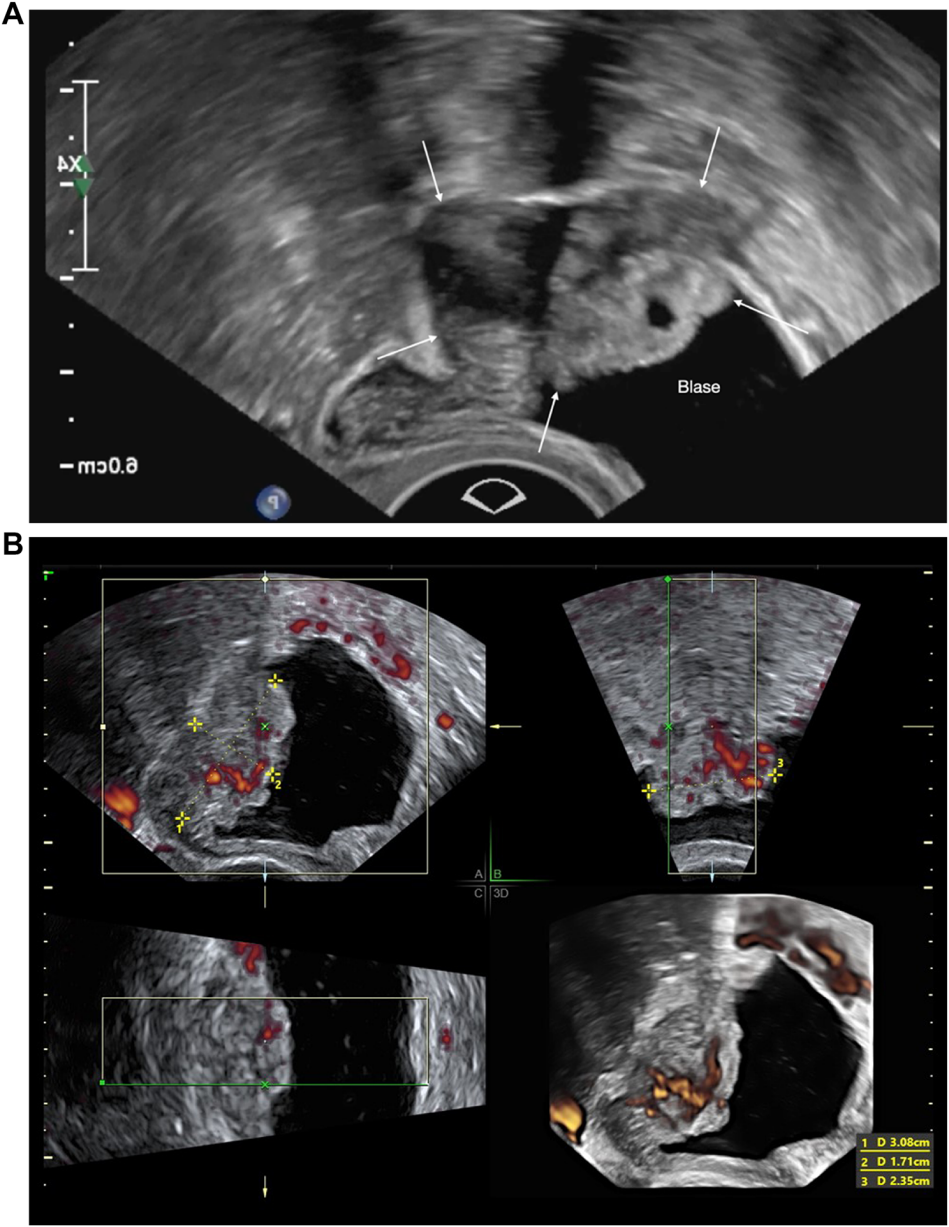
a Sonographic view of DE of the bladder (#Enzian(u)FB), presenting a full thickness defect at the bladder dome by a large inhomogeneous deep endometriosis nodule. b 3D demonstration of a severe bladder endometriosis (30.8 × 17.1 × 23.5 mm) originating from the posterior bladder wall (#Enzian(u)FB). A–C Multiplanar glass body demonstration (grey scale + color Doppler): A = sagittal view, B = transverse view, C = coronal view. 3D = surface demonstration of the cut plane with monochromic demonstration of the vascularization
